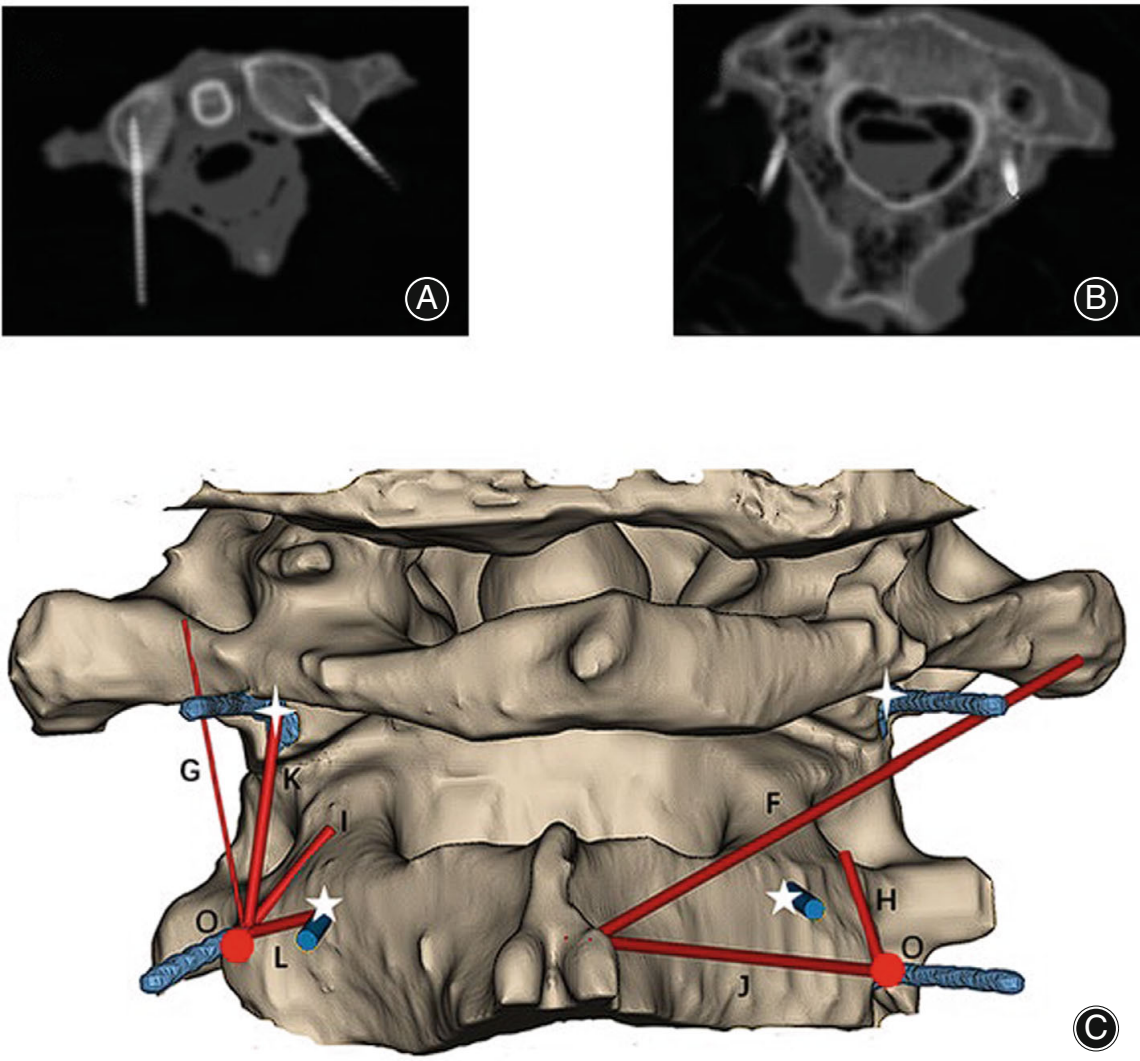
Axial CT scans and Mimics reconstruction images, red circles represent the anatomical reference point (O) marked by the kerf pins, white four‐pointed stars represent the C1 lateral mass screw placement points marked by the kerf pins, and white five‐pointed stars represent the C2 pedicle screw placement points marked by the kerf pins. (A) Axial CT shows the C1 lateral mass screw placement points marked by the kerf pins bilaterally; (B) the distance from C2 spinous process to C1 transverse process (F), the distance from the anatomical reference point to the superior edge of the posterior arch of the atlas (G), the distance from the anatomical reference point to the transverse foramen of the axis (H), the distance from the anatomical reference point to the spinal canal (I), the distance from the anatomical reference point to C2 spinous process (J), the distance from the anatomical reference point to C1 lateral mass screws (K), and the distance from the anatomical reference point to C2 pedicle screws (L) were measured.
